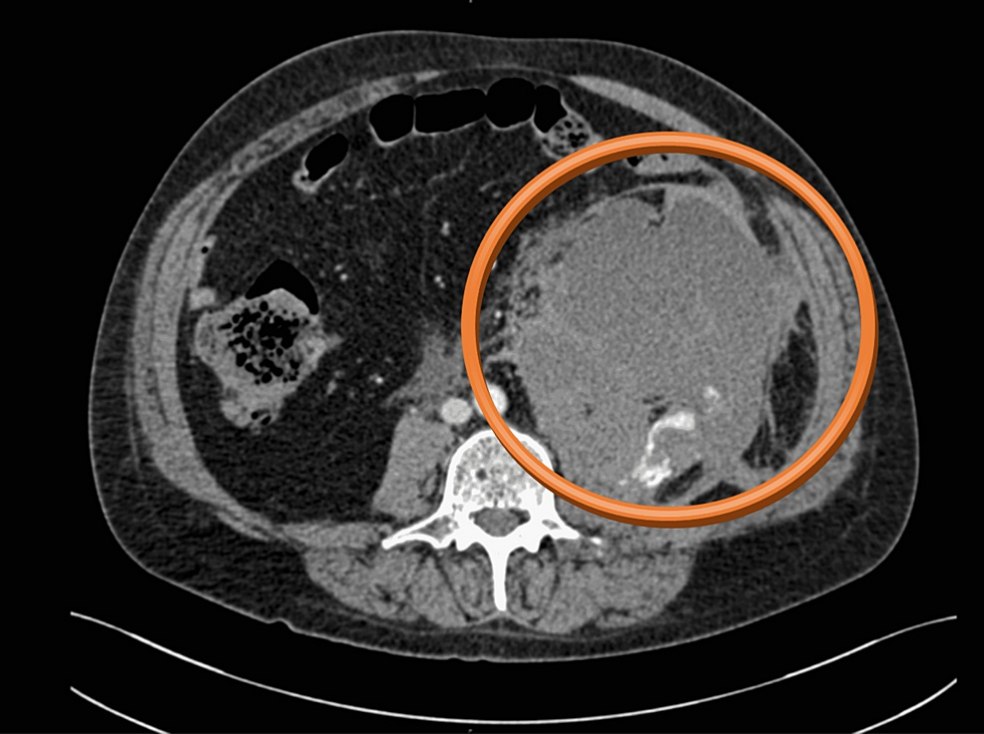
CT scan - axial plane The hematoma in the left retroperitoneum was of size 170 x 125 x 120 mm, attached to the left musculus psoas major, with present active bleeding
